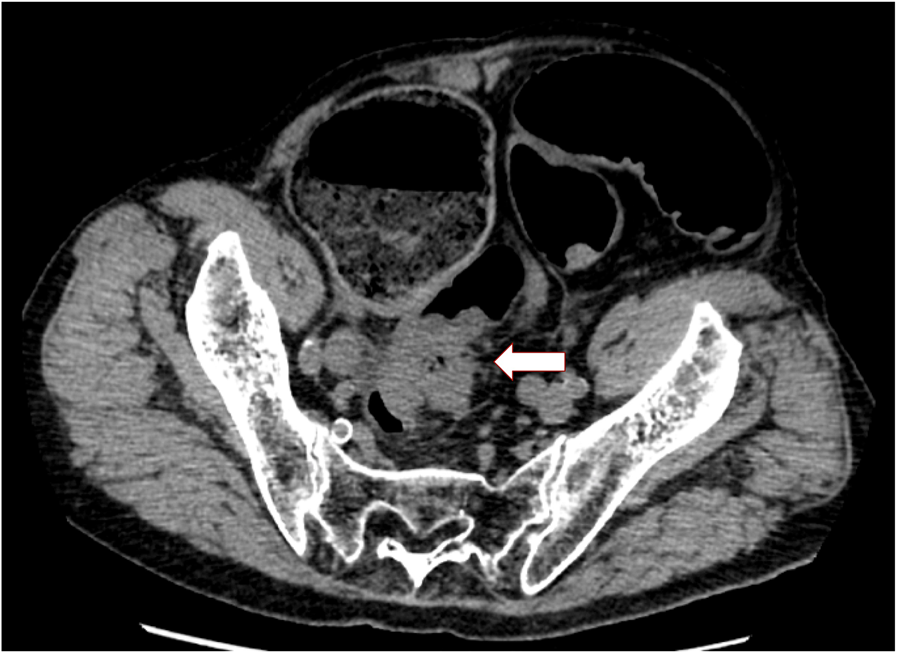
Abdominal multi-detector computed tomography scan. Rectal tumor (white arrow) and distended bowel on the oral side can be seen
